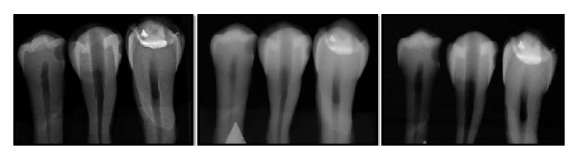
Example of X-rays taken using Kodak, Sirona, and Schick digital radiography systems.
